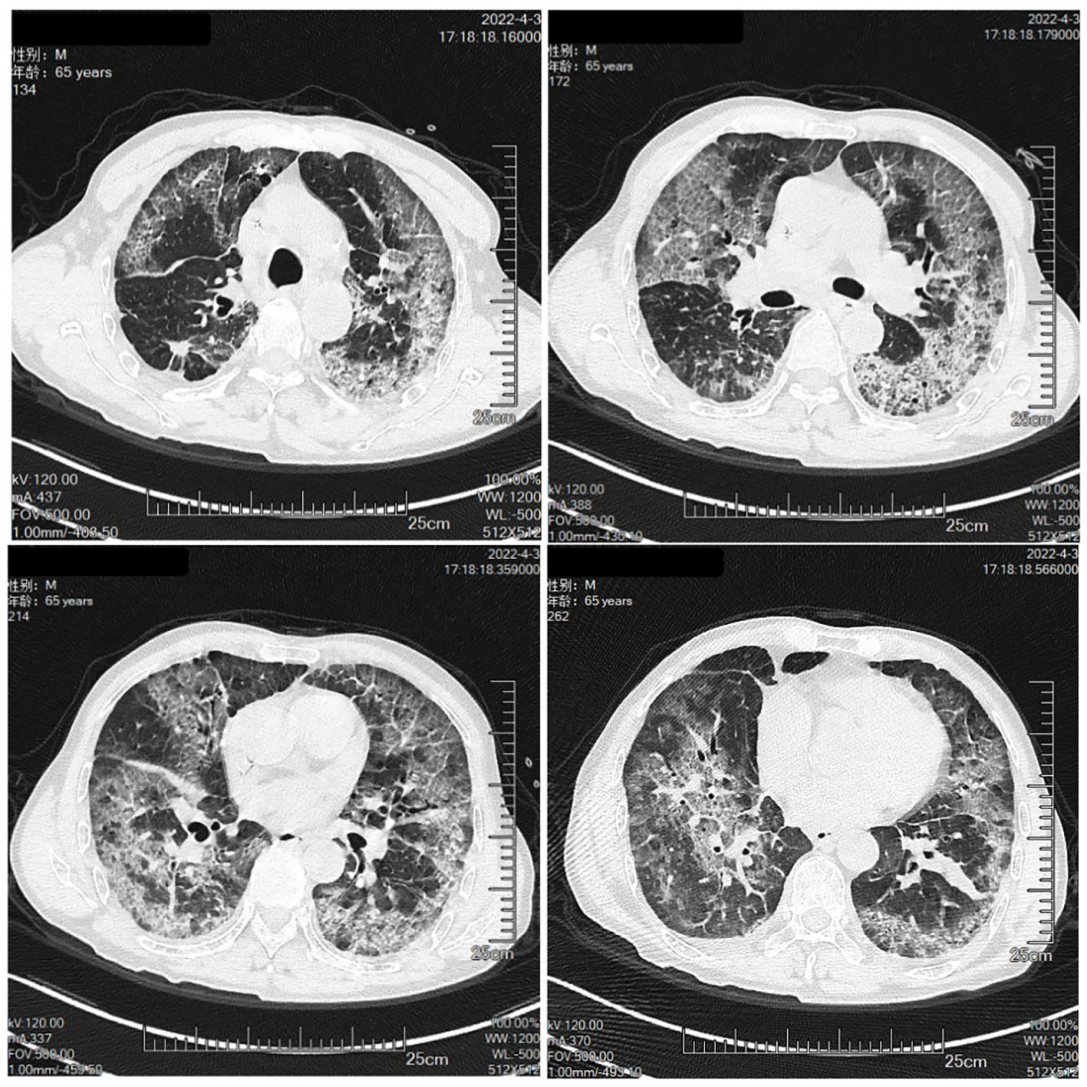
The Chest CT on April 3rd.
